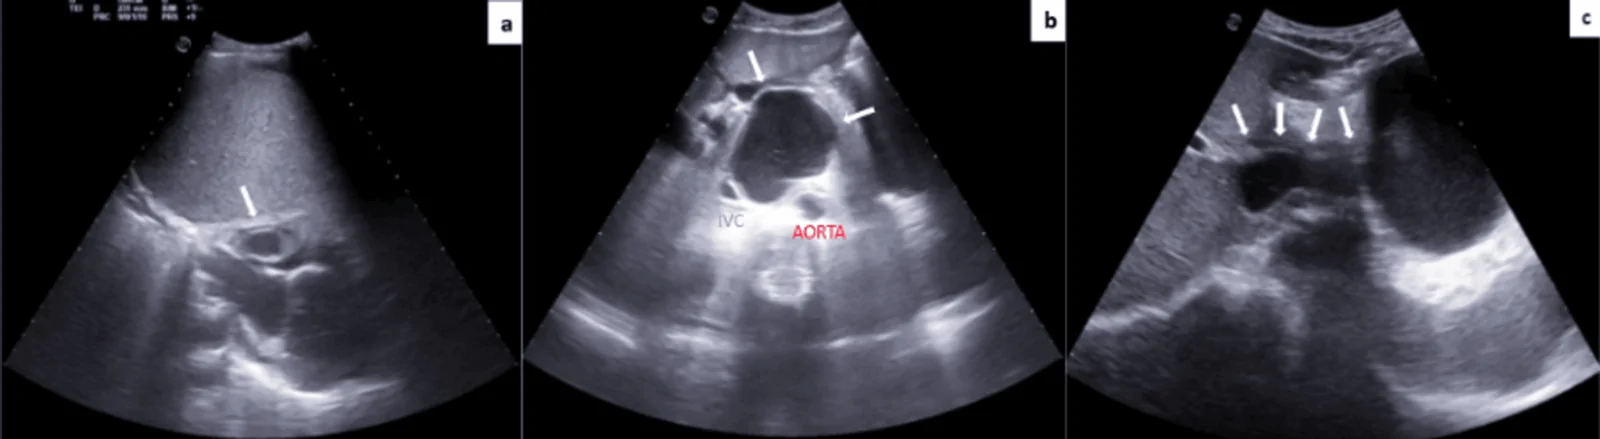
Focused ultrasound (abdominal and subxiphoid windows) (a) Thin-walled cystic structure (white arrow) within the right atrial cavity, subxiphoid four-chamber view; (b) Large abdominal collection (white arrows) in the interaortocaval space, adjacent to the hepatic inferior vena cava, subcostal transverse view; (c) The same collection extending superiorly along the course of the inferior vena cava, subcostal longitudinal view
